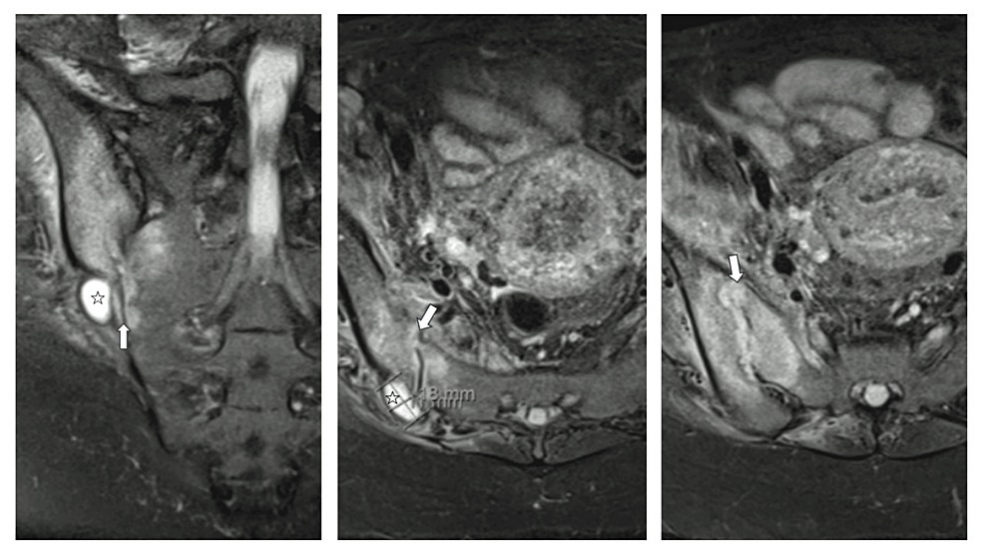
(Left to right) MRI of the sacroiliac joint showing an extensive right sacroiliitis and an adjacent abscess Inflammatory changes of the sacroiliac joint are marked with an arrow and the adjacent abscess is marked with a star.
